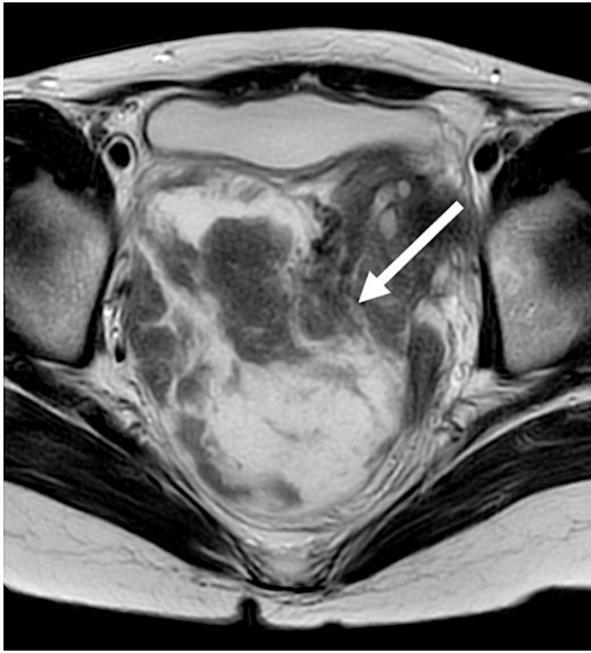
Axial magnetic resonance imaging revealed a 120 mm × 70 mm mass extending extensively into the retroperitoneum (arrow).
